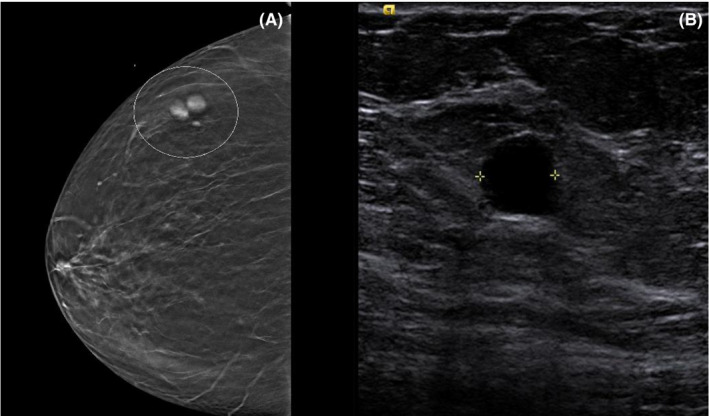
(A) A Cluster of radiopaque masses confined to superior lateral quadrant of right breast. (B) Ultrasonography image of the hypoechoic area that was biopsied and revealed the diagnosis of CLL/SLL
